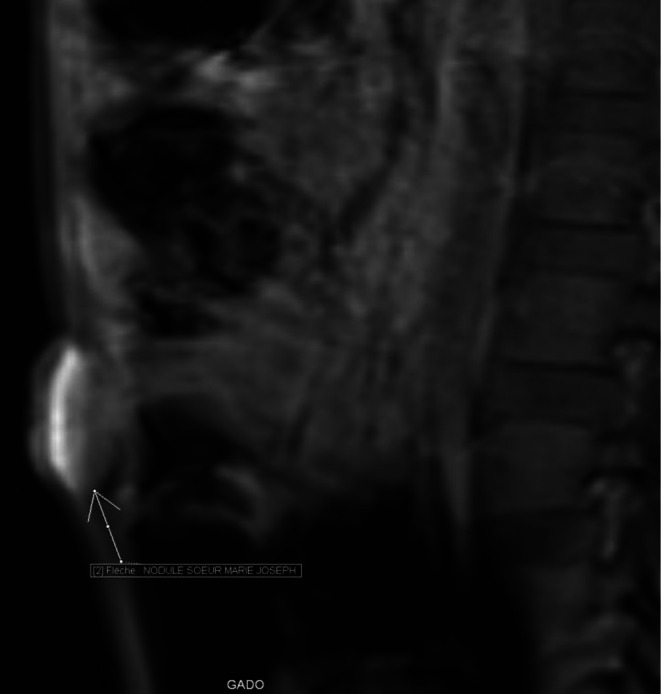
Abdominal MRI T1‐weighted Vibe fs sequence. Sagittal section showing an intensely enhancing umbilical tissue mass after injection of gadolinium contrast medium in connection with the Sister Mary Joseph nodule.
